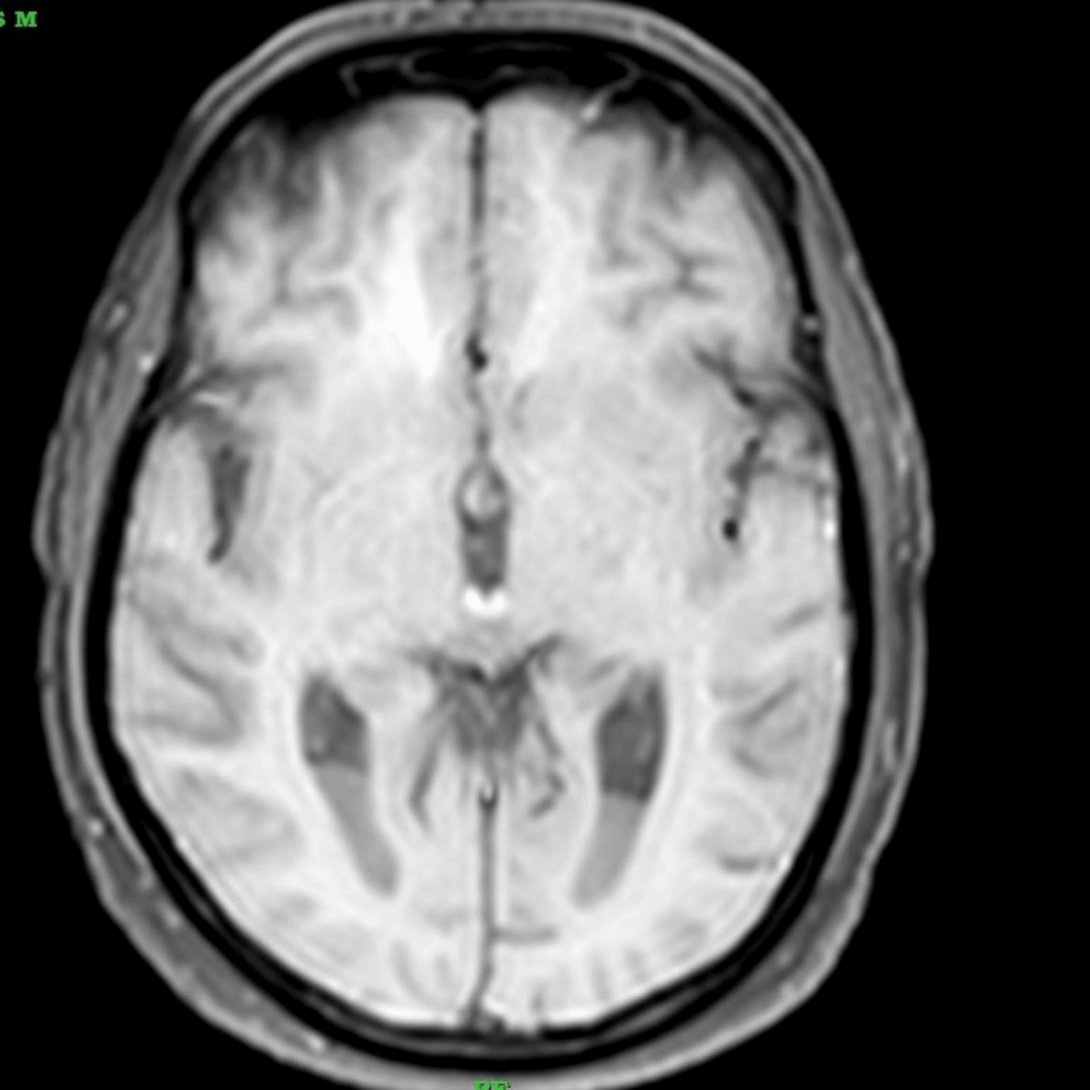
Axial T1-weighted MRI image shows isointense basal ganglia structures with relatively hypointense CSF, consistent with T1 characteristics
